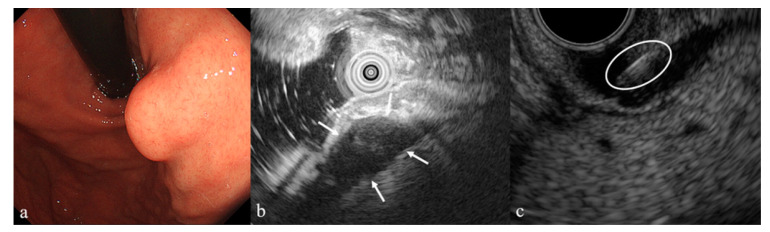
A 46-year-old man was referred to our department for evaluation of a gastric subepithelial lesion (SEL) measuring 15 × 9 mm, located in the cardia (a). Endoscopy revealed a rigid lesion with a negative cushion sign. Endoscopic ultrasound (EUS) showed a heterogeneous hypoechoic mass (white arrows) originating in the muscle layer (b), and computed tomography revealed an intraluminal growth pattern suspicious of gastrointestinal stromal tumor. Two needle (white circle) biopsies were obtained by endoscopic ultrasound-guided fine needle aspiration (EUS-FNA) (c); however, rapid on-site evaluation indicated that there were insufficient cells for cytologic examination. Our failure to obtain sufficient material, despite successful needle puncture, was probably attributable to the lesion’s small size and mobility. Thus, EUS-FNA has limited diagnostic accuracy for small SELs < 2 cm [1].
